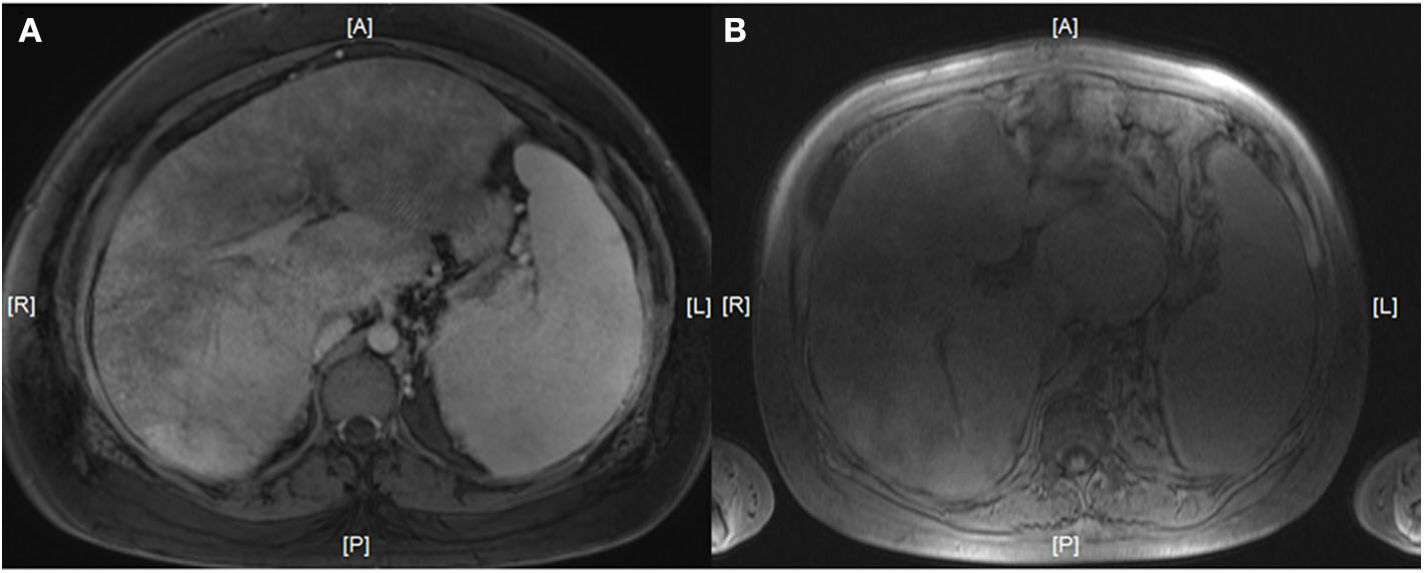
Magnetic resonance imaging showing a (partial) Budd-Chiari syndrome in two PNH patients. Patient 2 (A, gestational week 22) and 3 (B, gestational week 25). A, anterior; L, left; P, posterior; R, right.
